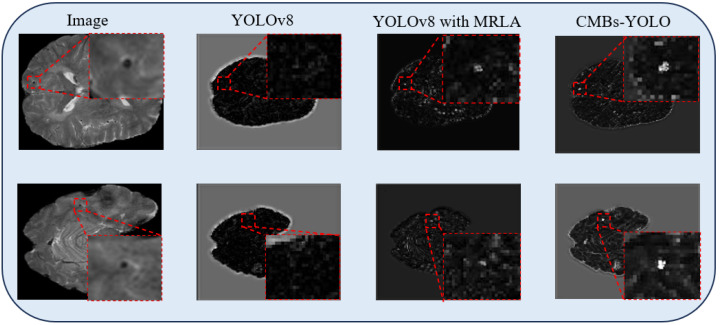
Feature responses of CMBs in MRI.
